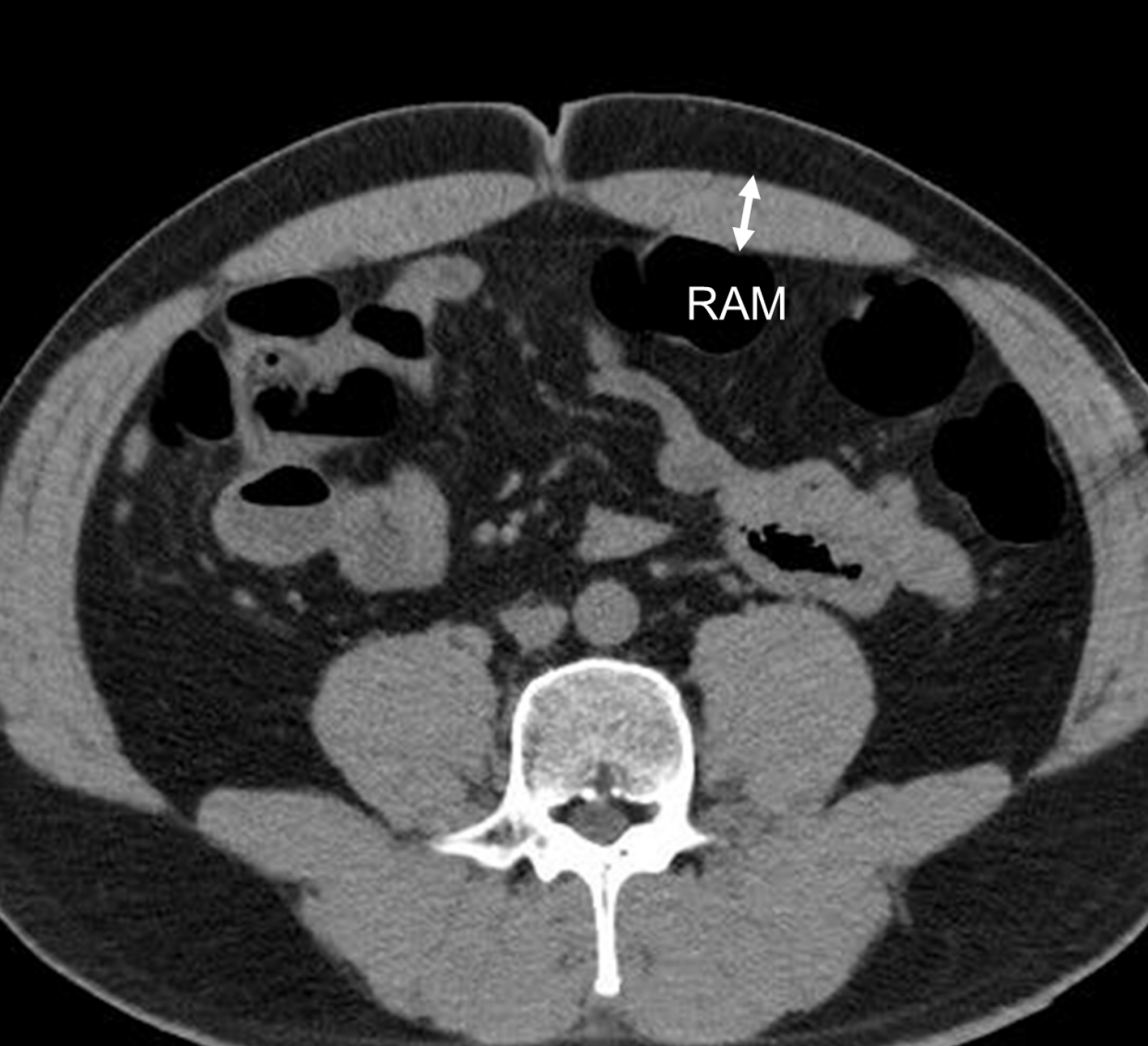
The thickness of the rectus abdominis muscle. The thickness of the rectus abdominis muscle were measured to the nearest 0.01mm from CT images with the picture archiving and communications system (PACS) viewer program (Marosis m-view 5.4). RAM, thickness of the thickest part of the rectus abdominis muscle thickness at the umbilicus level.
